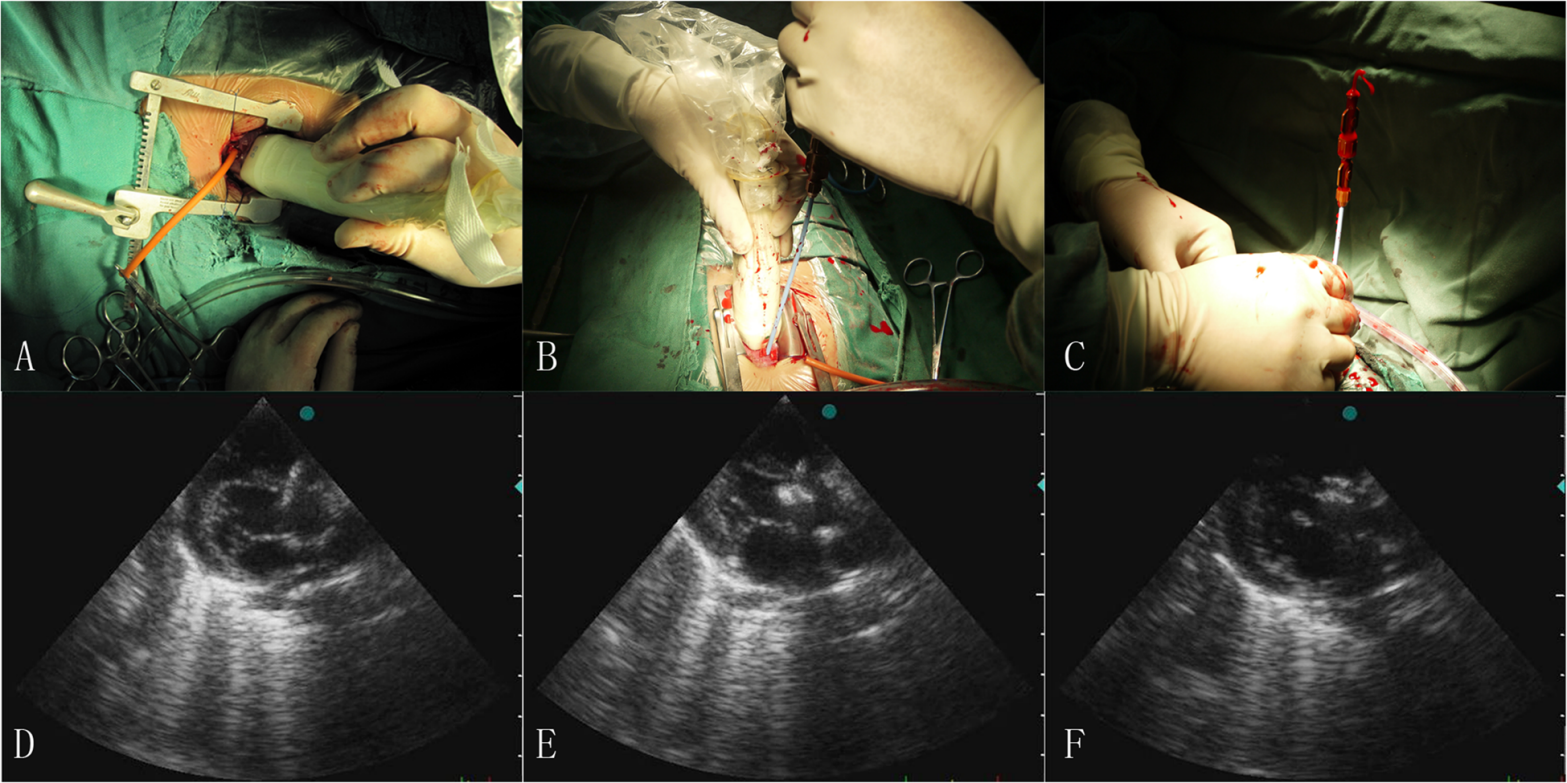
The steps of periventricular device closure of perimembranous VSD. (a) A trans-epicardial echocardiography view after a small stenotomy. (b) A surgeon handles the guide-wire under the guidance of trans-epicardial echocardiography. (c) Blood launching from the delivery sheath after it passed through the defect in to the left ventricle. (d) A right disc was deployed at first step. (e) A device was completely deployed and the delivery sheath will be kept for 5 min. (f) A device was successfully deployed without any complications
